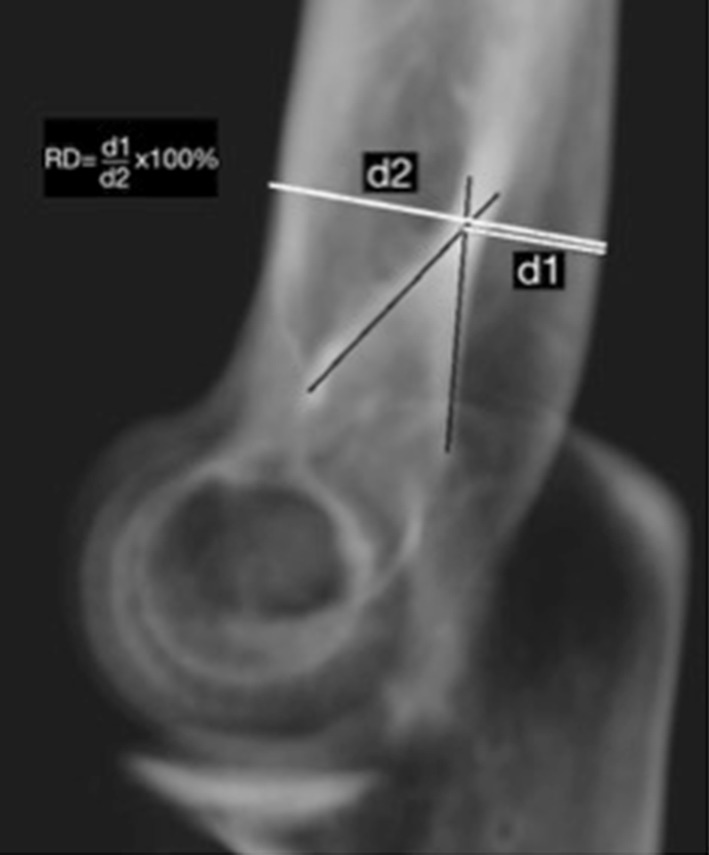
Digitally reconstructed radiograph (DRR) of the humerus in lateral view, depicting how the relative distance (RD) of the humerus is measured. The RD is defined as RD = (*d*1/*d*2) × 100%, with *d1* the distance from the dorsal side of the humerus to the projection of the posteromedial cortex (measured at the intersection point of the cortical lines, as represented by the intersection of the drawn black lines in the figure) (mm), and with *d2*, anteroposterior diameter of the humerus (mm). The *lines d1* and *d2* are measured perpendicular to the bone axis. Finally, the RD is calculated as the length ratio of *d1* and *d2* and expressed as a percentage
